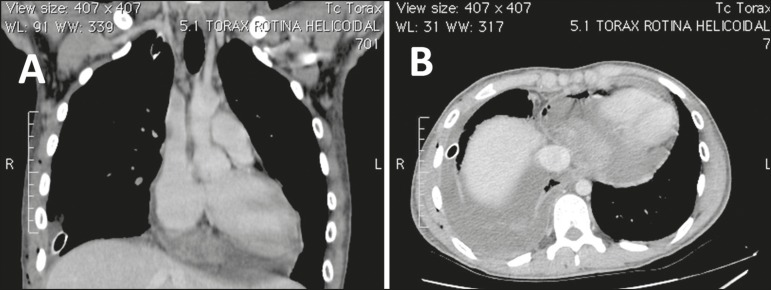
Contrast-enhanced chest CT. Coronal (A) and axial
